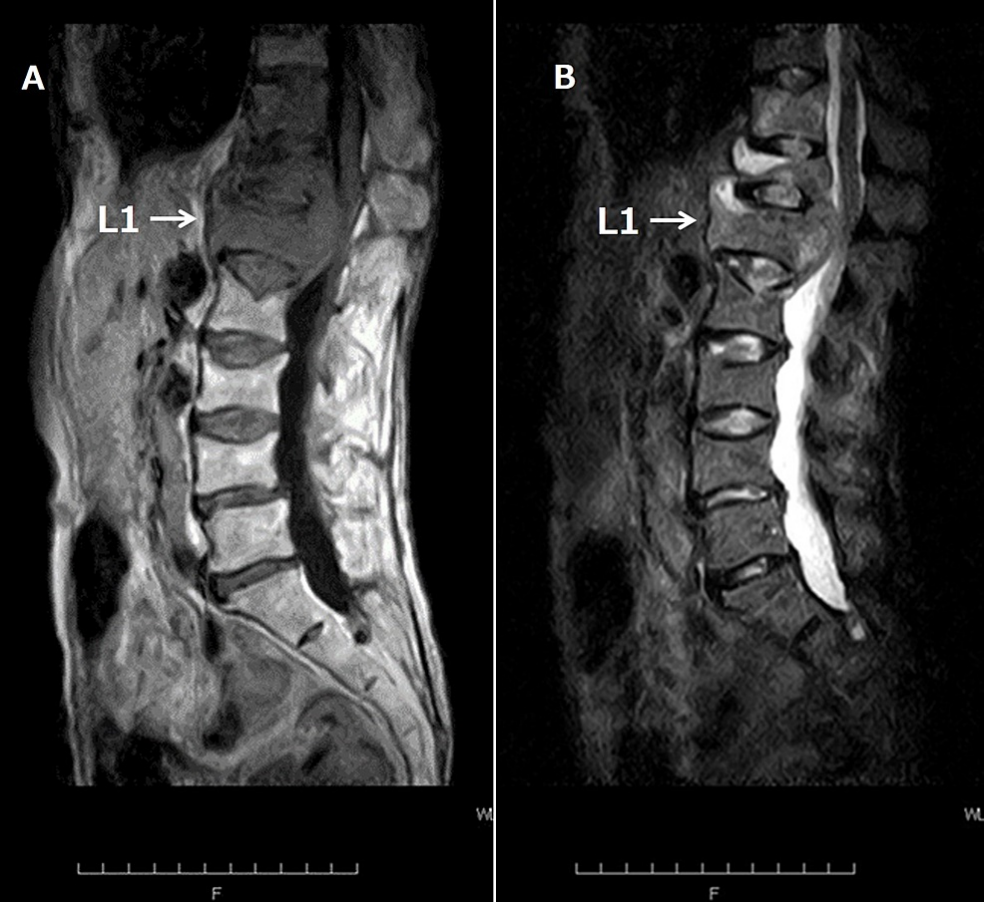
Lumbar magnetic resonance sagittal imaging T1-weighted image (A) and short tau inversion recovery image (B) on admission. (A) T12-L1 has low-intensity lesions. The bone lesions are strongly compressing the dural sac posteriorly and narrowing the spinal canal. (B) T12-L1 has high-intensity lesions. The bone lesions are strongly compressing the dural sac posteriorly and narrowing the spinal canal. T12: Thoracic vertebra 12; L1: lumbar vertebra 1
